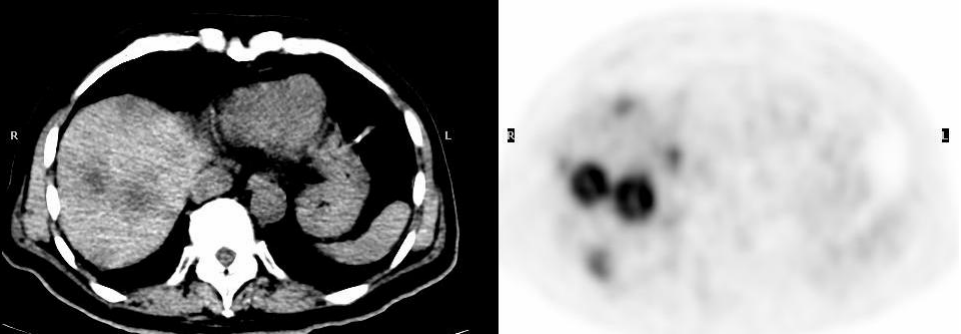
FDG PET-CT images demonstrate multiple necrotic hepatic metastases while bone scan and CT two months earlier were negative.
